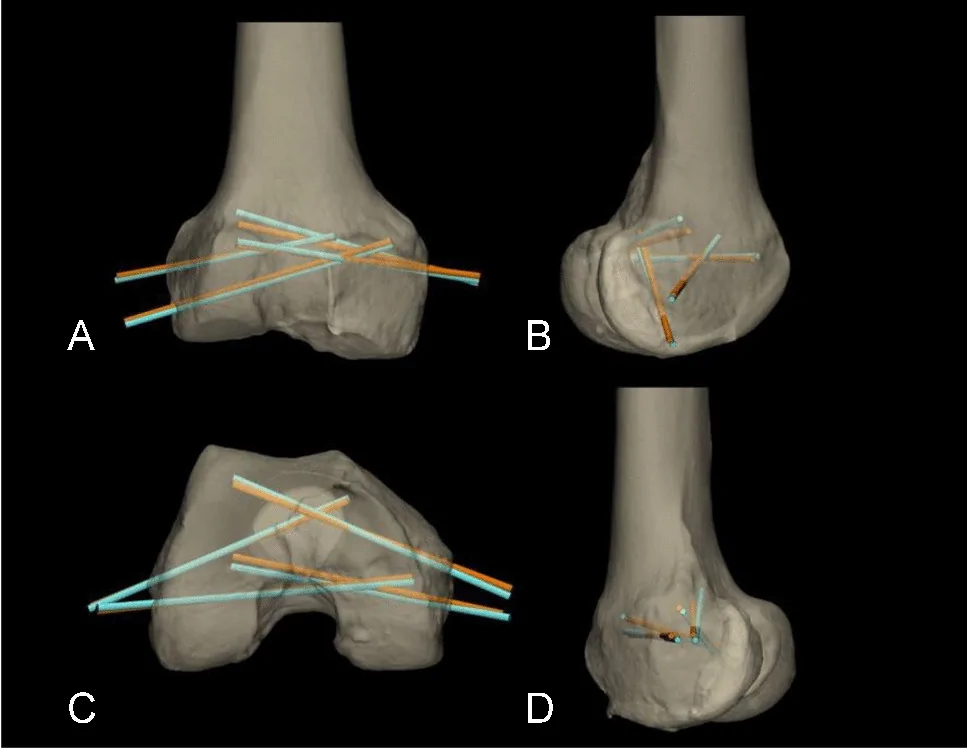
Superimposition of 3D reconstruction of pre- and post-operative CT scans. A Coronal, B lateral, C axial, and D medial view. Angular deviation between planned (blue) and actual (orange) tunnels
